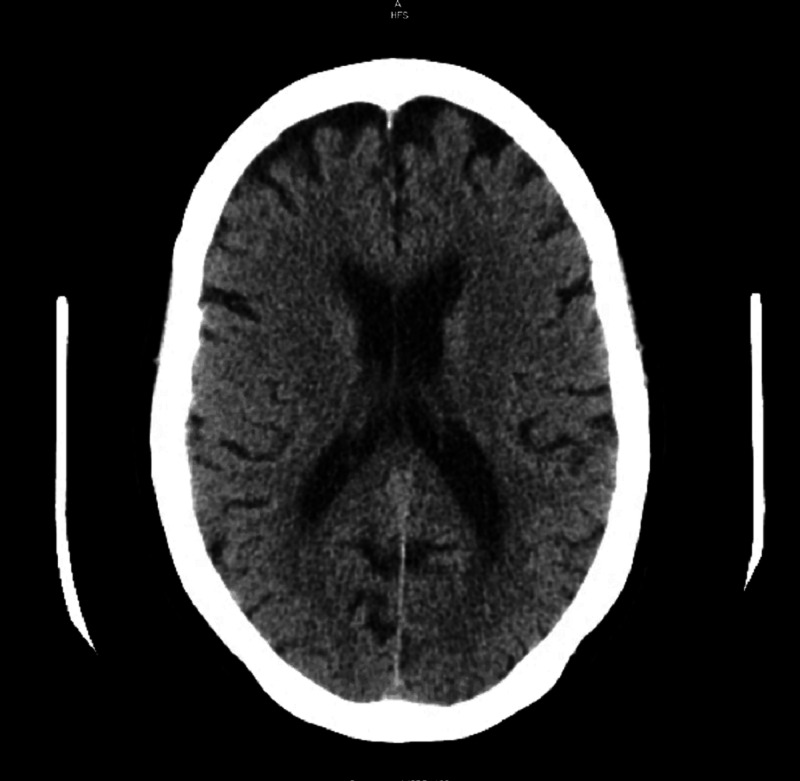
Computed tomography Scan of Head with out contrast showed no acute abnormalities.
